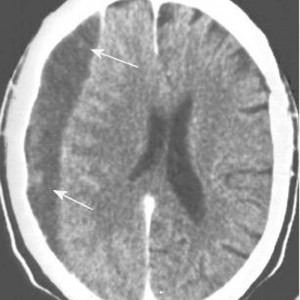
Chronic subdural haematoma of right brain hemisphere (CT scan); what should be noted is the hypodense aspect of the haematoma and the mass effect it has on the structures of the median line
